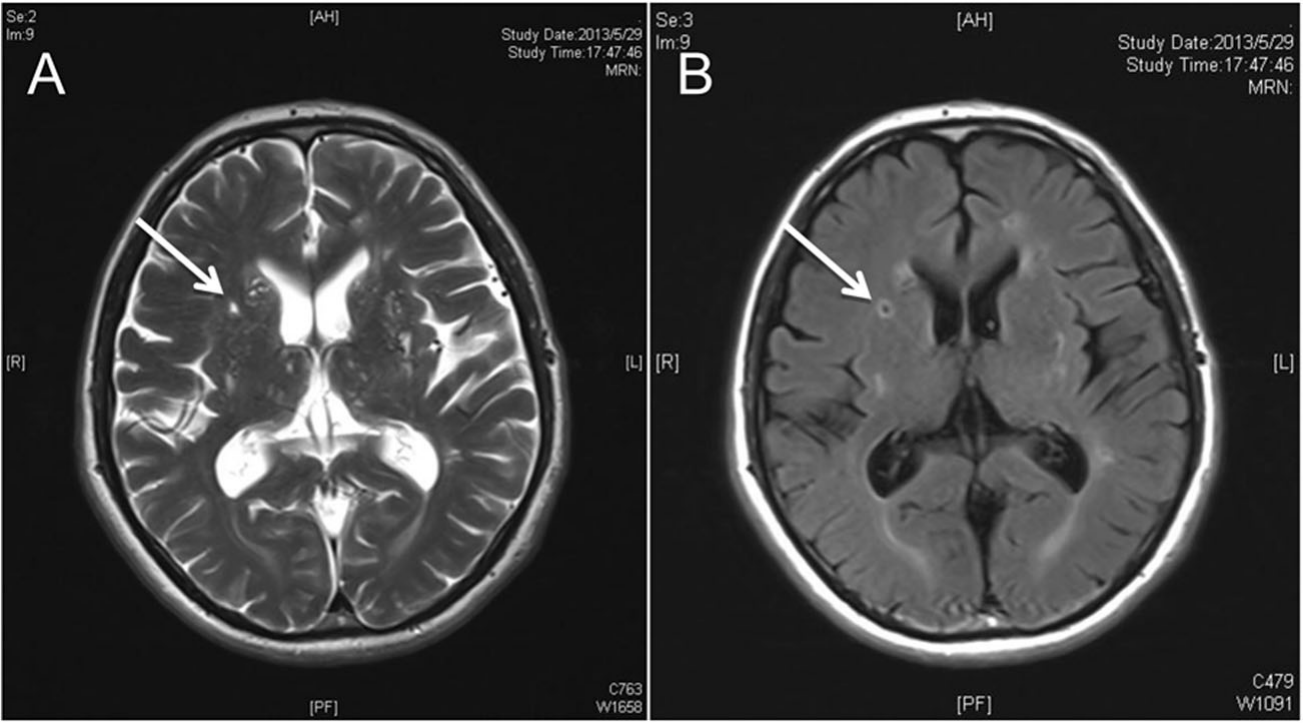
a Axial cranial T2 weighted MRI shows a lacunar infarct lesion of hyperintensity (*white arrow*). b Axial cranial FLAIR shows a lacunar infarct lesion with ring of hyperintensity and core of hypointensity (*white arrow*)
